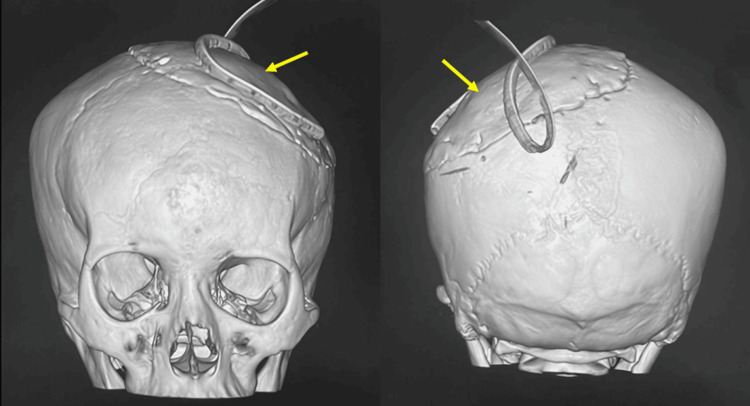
Postoperative 3D CT scan showing the location of the cranioplasty (arrow).
